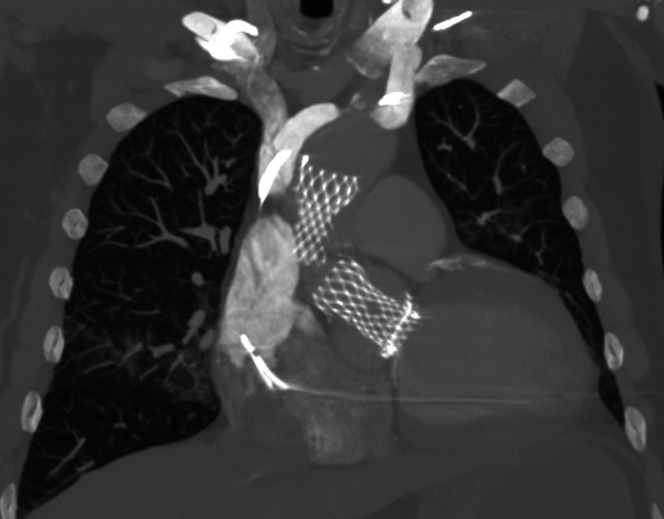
Computed Tomography of Chest Coronal view of the new 26-mm Evolut FX+ transcatheter aortic valve replacement valve implanted status post snare-mediated repositioning of the original valve in ascending aorta distal to the brachiocephalic artery.
